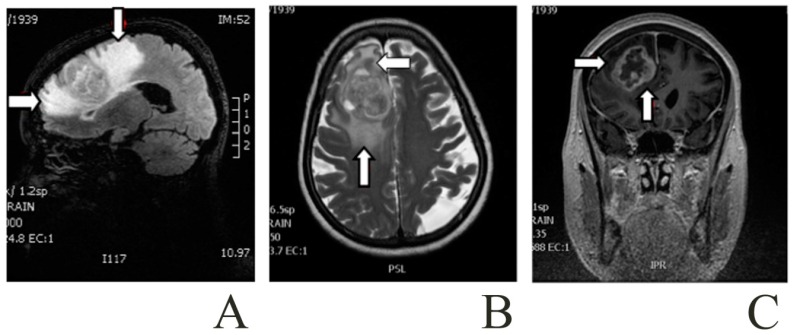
Patient with a right frontal glioblastoma and a marked area of peritumoral edema. Images from a Brain Magnetic Resonance. The arrows point to the peritumoral edema close to the glioma: (A) Sagittal T1-weighted; (B) Axial T2-weighted; and (C) Coronal T1-weighted contrast-enhanced with gadolinium.
